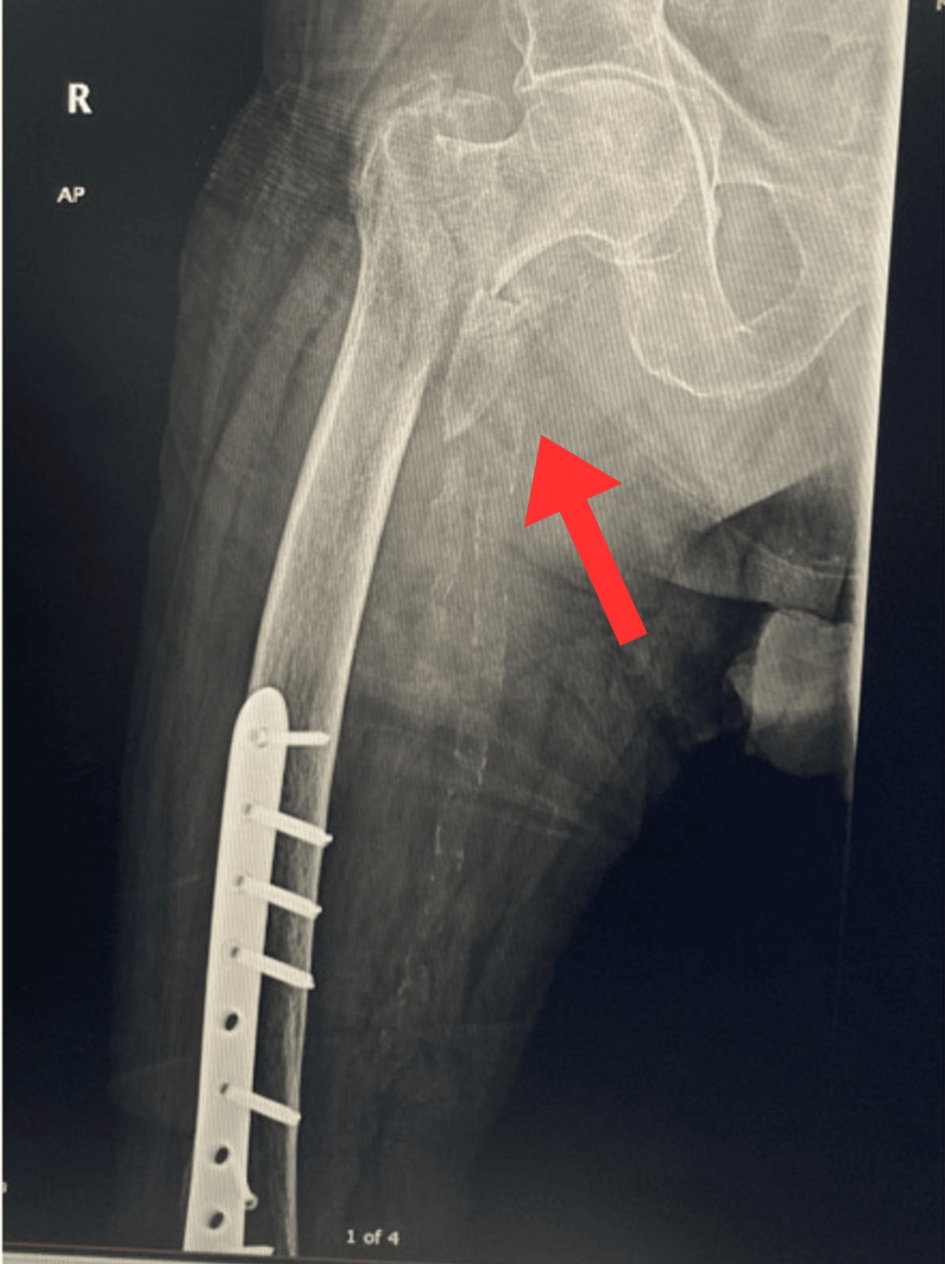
Preoperative X-ray of the right hip (AP view), 25 May 2024, demonstrating a comminuted displaced intertrochanteric fracture of the right proximal femur.
